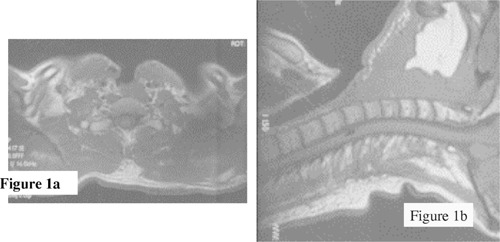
Transverse and sagittal magnetic resonance images views of a stoma and the surrounding anatomy.
